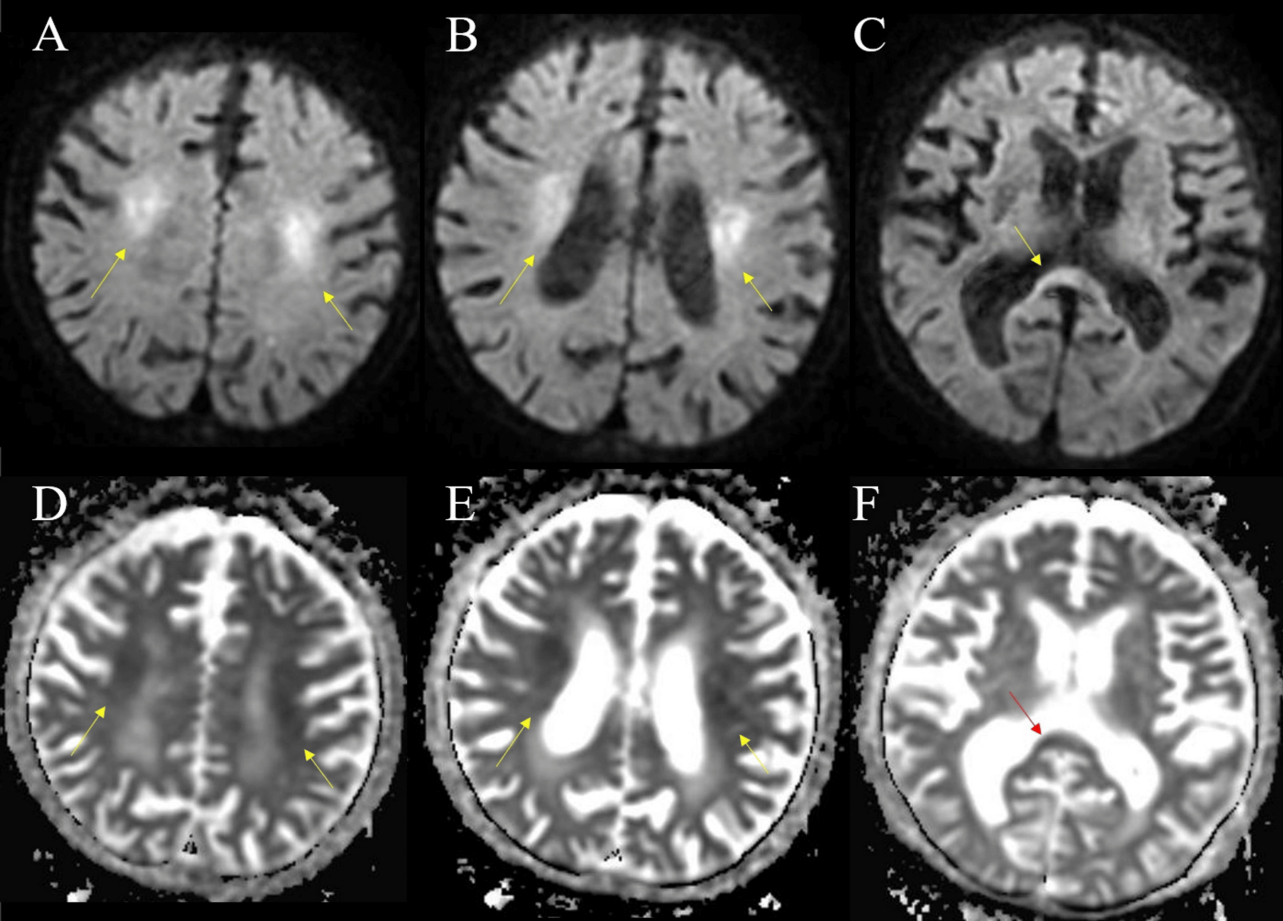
The results of brain magnetic resonance imaging (MRI) on admission. (A-C) Diffusion-weighted brain MRI showing hyperintensity in the splenium of the corpus callosum (SCC) and the bilateral deep white matter symmetrically (arrowheads). (D-F) Apparent diffusion coefficient (ADC) values were decreased in the areas of hyperintensity by diffusion-weighted brain MRI (arrowheads).
